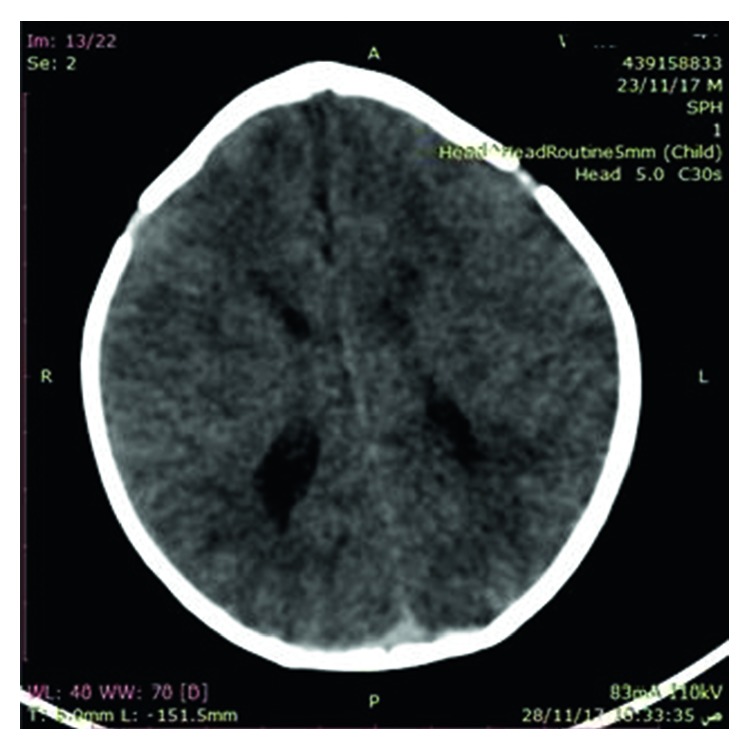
CT showed metopic suture craniosynostosis.
